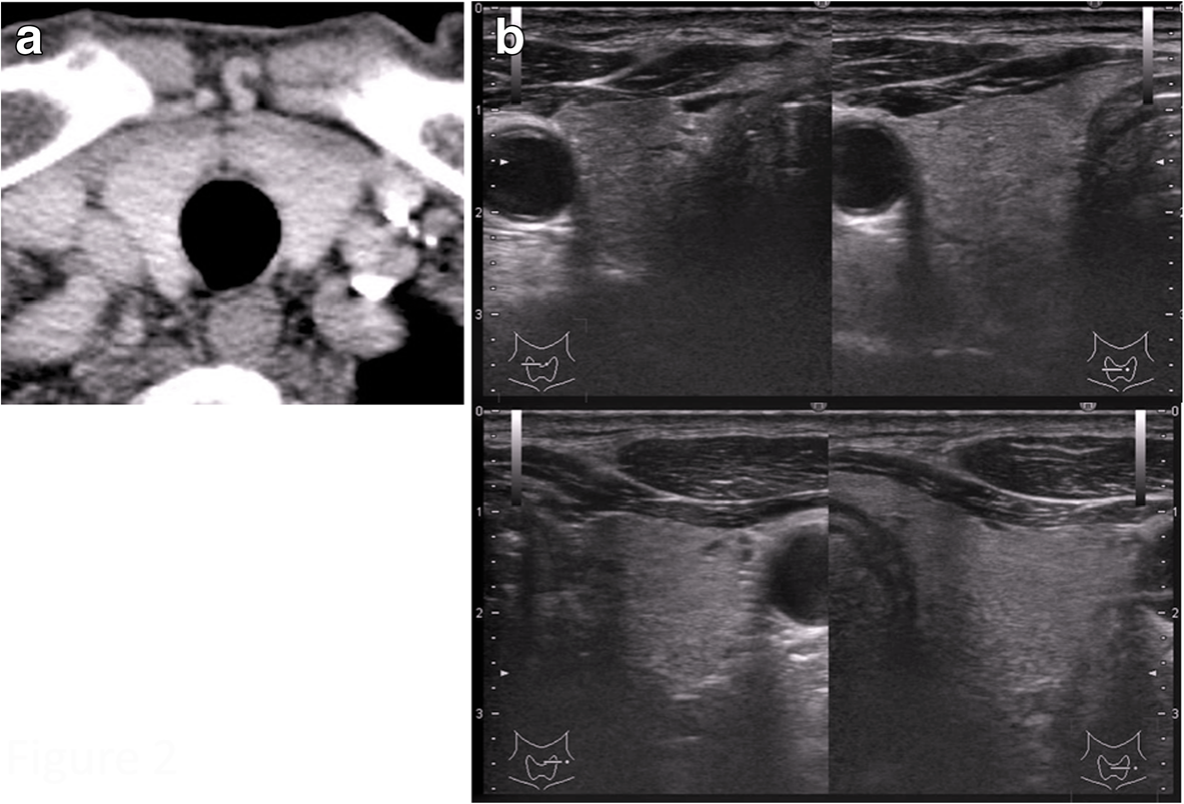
Computed tomography (a) and ultrasound (b) of the thyroid showed no abnormal findings
